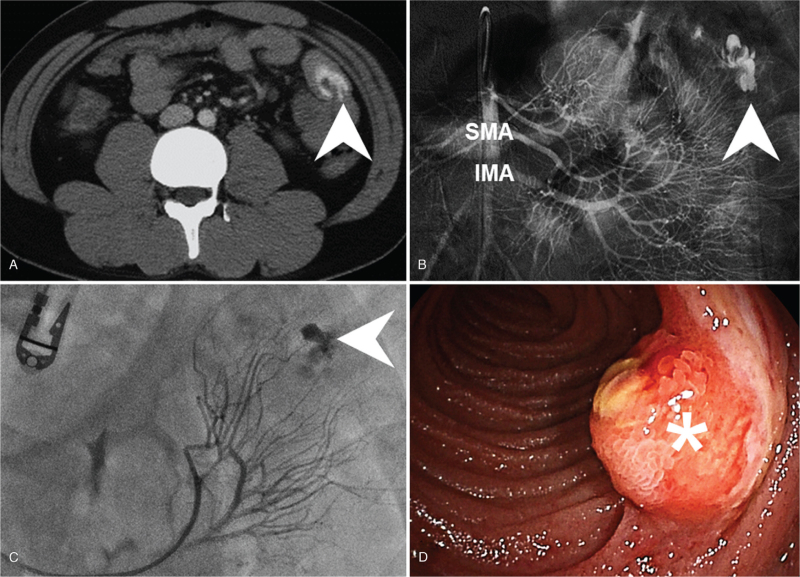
Active contrast extravasations and segmental wall thickening of jejunum (arrowhead) detected by computed tomography of abdomen with biphasic contrast enhancement (A). Emergent transcatheter arterial embolization performed immediately and after angiographic confirmation of active bleeding from branch of superior mesenteric artery (B) superselective embolization completed by gelform cubes injection (arrowhead) (C). A 15 millimeters subepithelial tumor at proximal jejunum (asterisk) with ulceration centrally and hyperemia peripherally observed via double balloon enteroscopy (D). SMA: superior mesenteric artery, IMA: inferior mesenteric artery.
